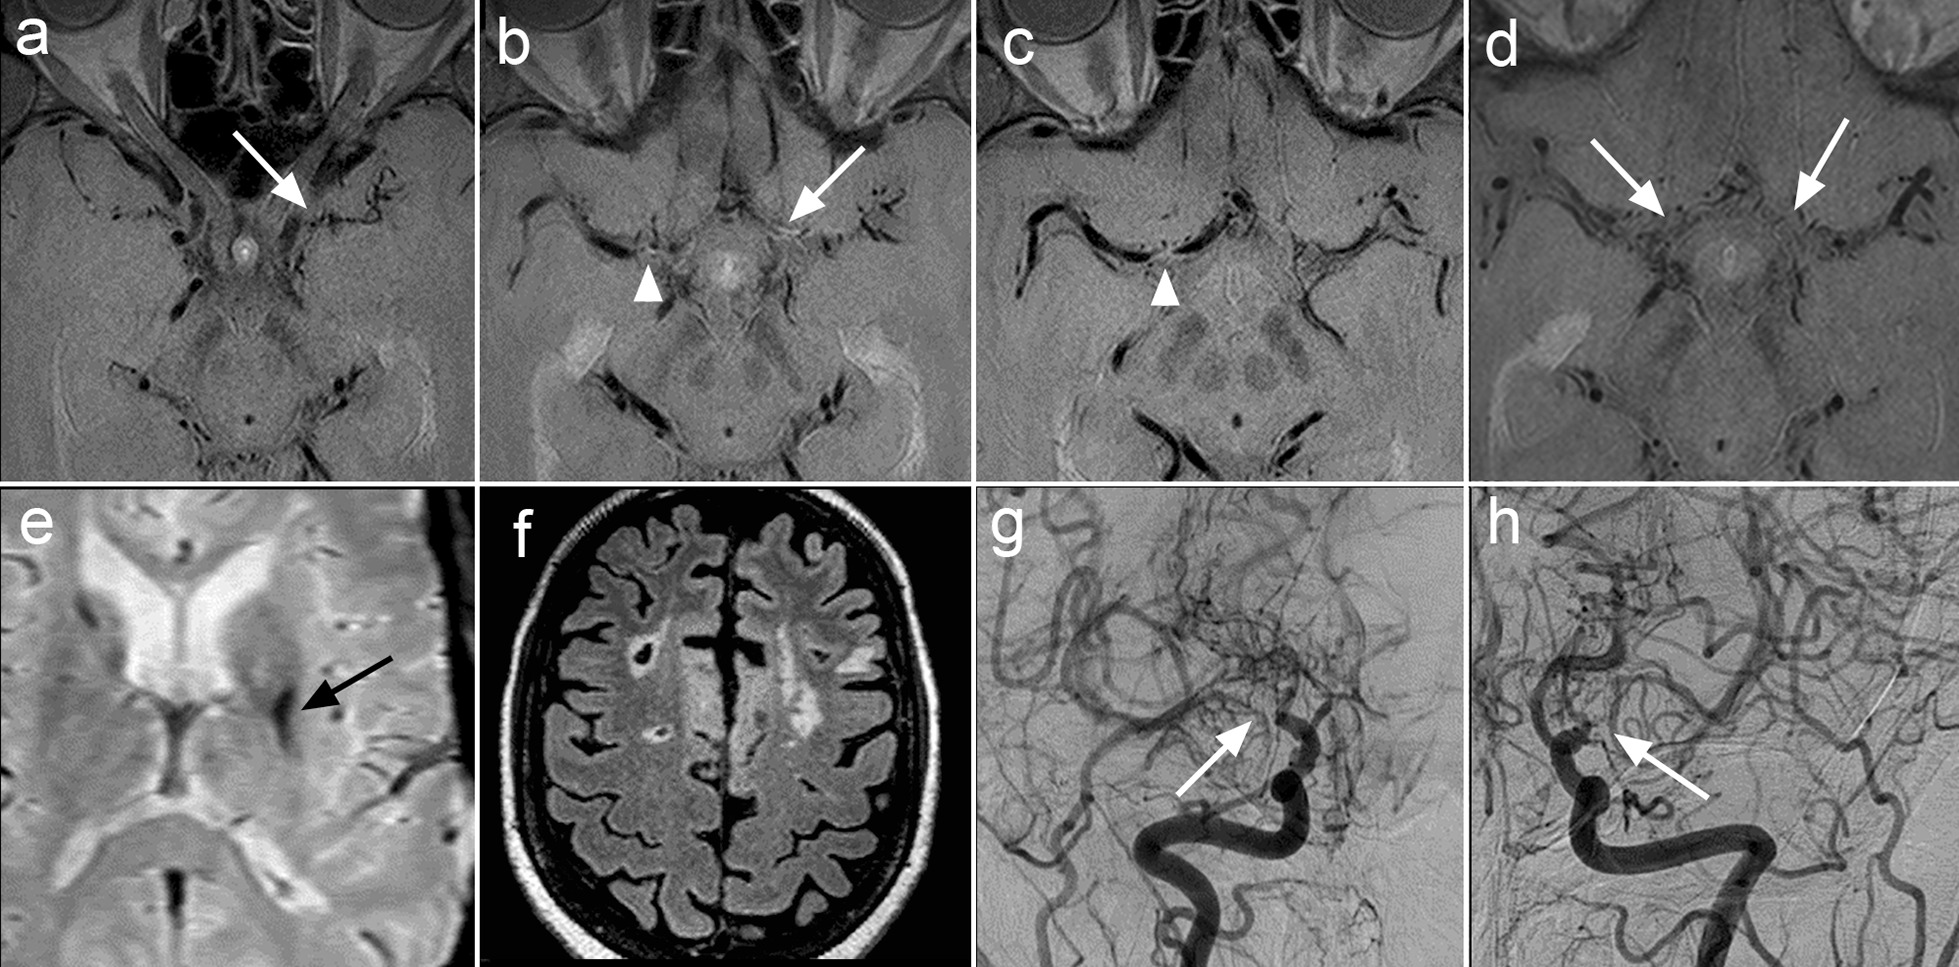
Variable pattern of vessel wall enhancement among different affected segments in a single patient. A 47-year-old female with a 1-year history of recurrent infarcts in the bilateral MCA territories had a history remarkable for a heterozygous factor V Leiden mutation. Otherwise, the patient had no laboratory findings of hypercoagulability (including protein C, protein S, antithrombin III, antiphospholipid antibody). No vascular risk factors, such as smoking, hyperlipidemia/hypercholesterolemia, hypertension, or diabetes. A trans-esophageal echocardiogram was unremarkable. Rheumatologic history and laboratory work-up were also unremarkable with no evidence of vasculitis or autoimmune disorder. Axial VWI demonstrates narrowing and negative remodeling of the supraclinoid left ICA without vessel wall enhancement (white arrow, a), while there was grade I circumferential wall enhancement of the distal left ICA (not shown). There was also eccentric grade I enhancement of the posterior wall of the distal right ICA (white arrowhead) as well as grade I circumferential wall enhancement of the left A1 segment at the same level (white arrow, b). The left M1 segment demonstrates negative remodeling throughout without vessel wall enhancement (white arrow, c). There is marked focal negative remodeling at the junction of the right M1 and A1 segments with faint grade I enhancement (white arrowhead) and sparing of the mid to distal right M1 segment and distal right A1 segment (c). Two-year follow-up VWI for descriptive purposes showed that there were no identifiable remnants of the terminal ICAs, proximal M1 or proximal A1 segments (white arrows) (d). An axial gradient echo image shows chronic blood product in the left lentiform nucleus (black arrow, e). An axial T2 FLAIR image shows chronic infarcts of the bilateral cerebral hemispheres (f). Right frontal (g) and left frontal (h) DSA images (CCA injections) further show the bilateral areas of stenosis and proliferation of lenticulostriate vessels, more marked on the right
